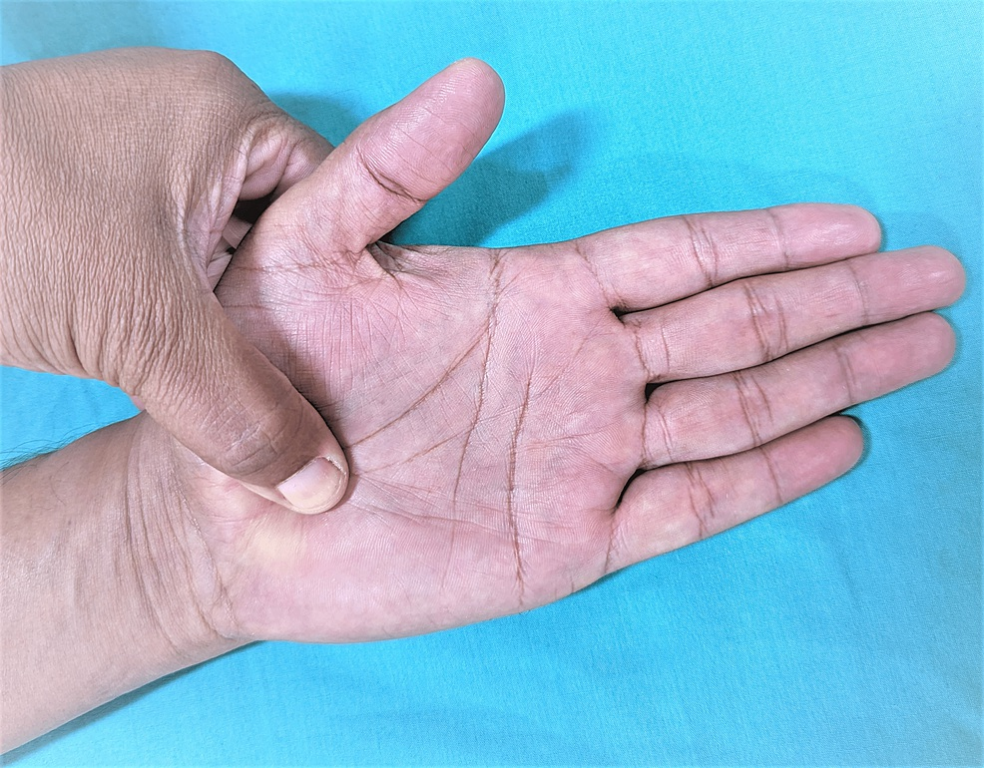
Median nerve compression test This is an original image from the ultrasound lab department of the American University of Antigua
